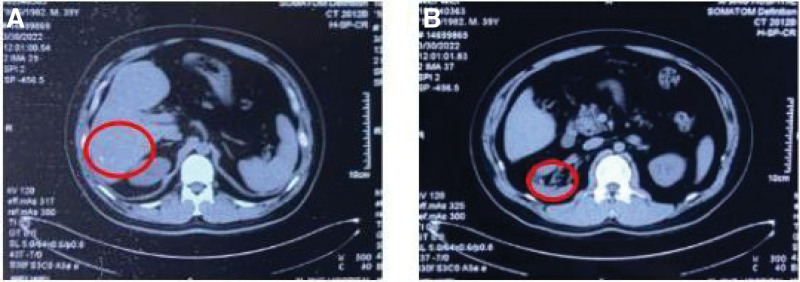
The results of a plain CT scan of the liver and kidney were followed up 5 years after the operation. (A) The results of a plain CT scan of the liver and kidney were followed up 5 years after operation. (B) Plain CT scan of the right kidney showed a patchy low-density shadow. CT = computed tomography.
